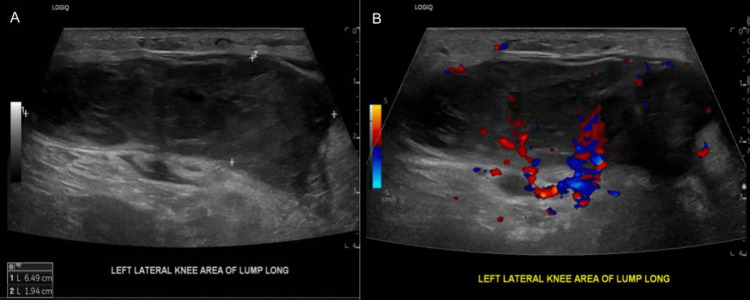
Ultrasound scan of the lump above left lateral knee area A) longitudinal view showed a heterogeneous hypoechoic mass, B) longitudinal view with Doppler study where the neovascular formation was visualized.
